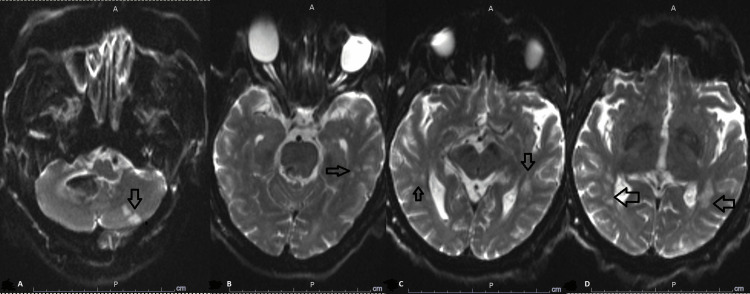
Subsequent diffusion trace MRI brain Diffusion trace MRI brain showing a new left-sided cerebellar infarction (arrow in A) with new punctate bilateral infractions affection cerebral hemispheres (arrows in B, C, and D)
